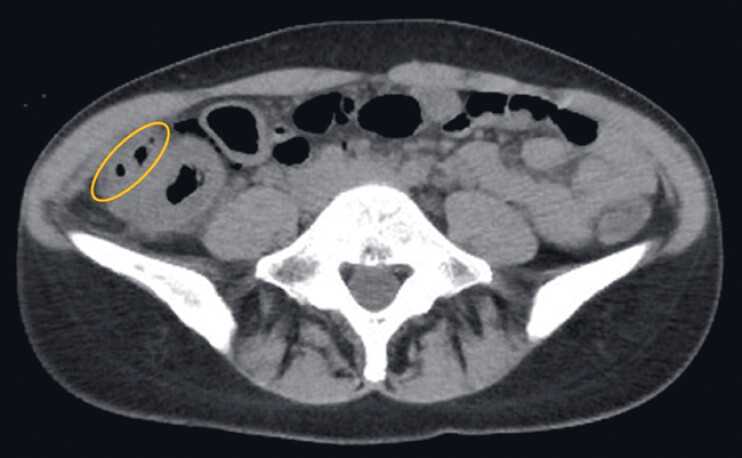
Computed tomography showing a small amount of extraintestinal air (yellow oval) around the ulcer site after endoscopic submucosal dissection.
